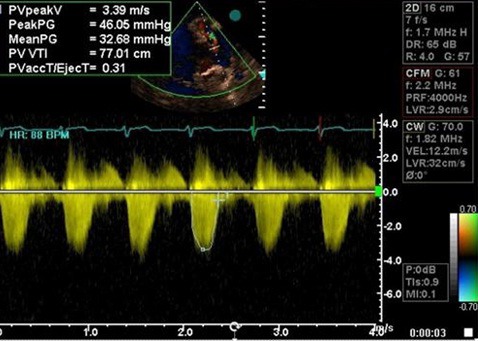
CW Doppler study across pulmonary valve showing mild to moderate valvular PS (PPG=46 mmHg).
